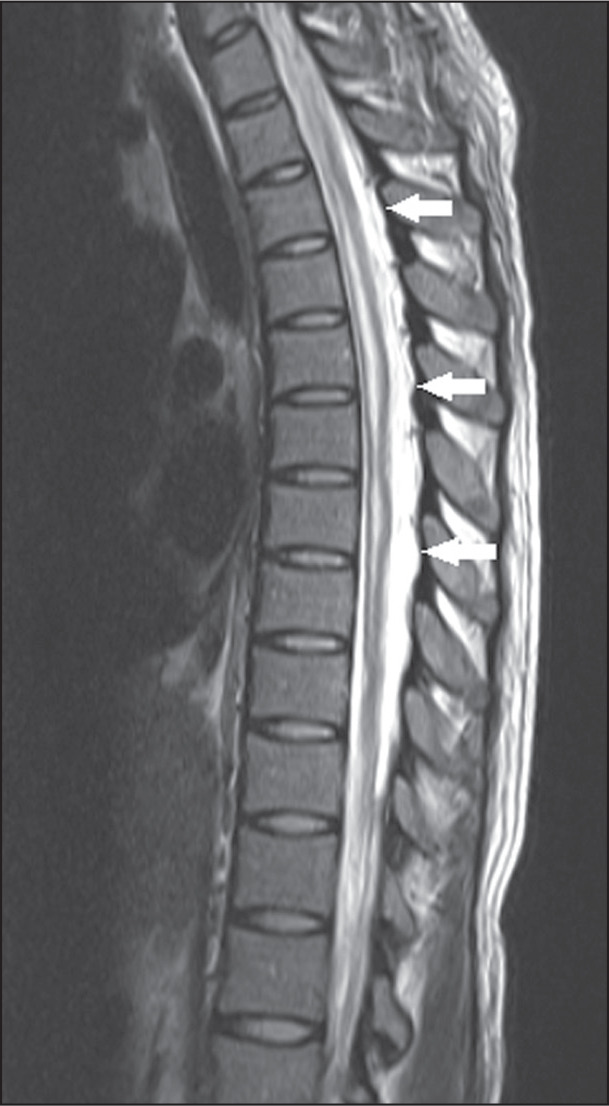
Zoster myelitis. Sagittal T2-weighted MRI sequence showing marked spinal
